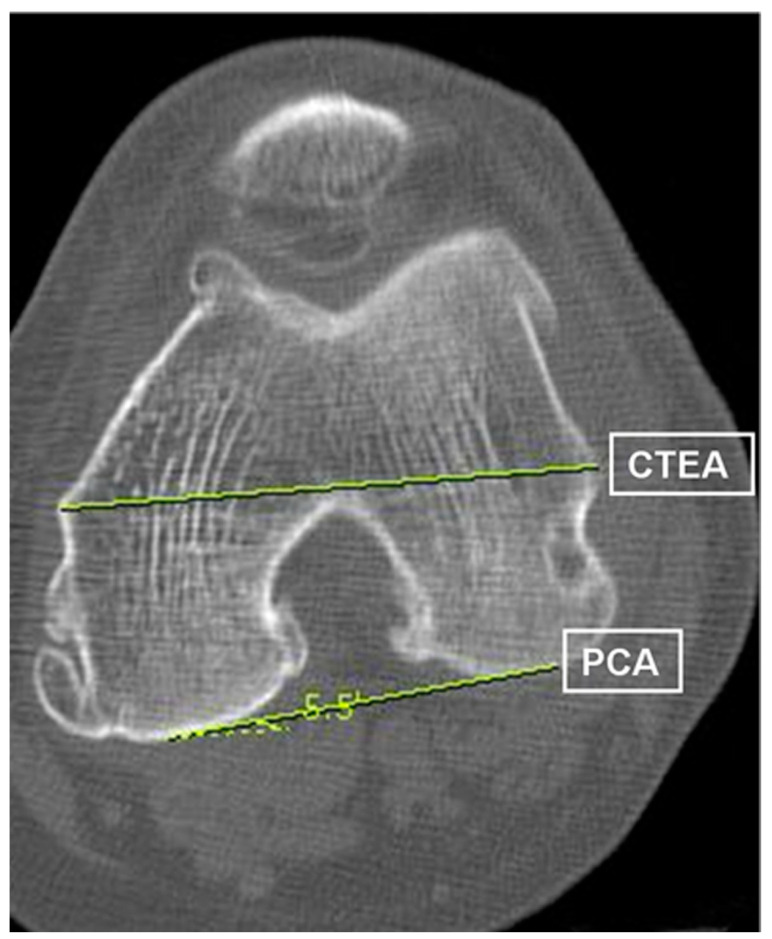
Angles between clinical transepicondylar and posterior condylar axes measured on the CT images are defined as preoperative femoral rotation angle on CT (TEA-PCA). CT, computed tomography.
